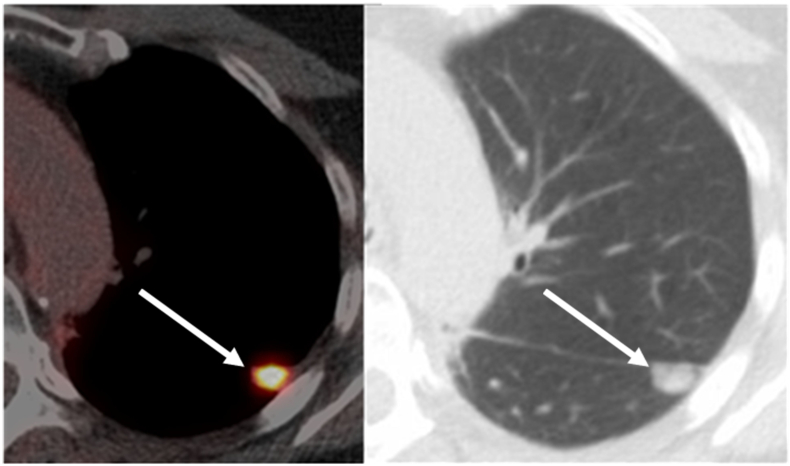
A ^18^F-FDG PET/CT scan 6 months after RAMIE demonstrating a 2.0 cm pulmonary metastasis in the left upper lobe with pathologic FDG uptake (indicated by the arrow).
